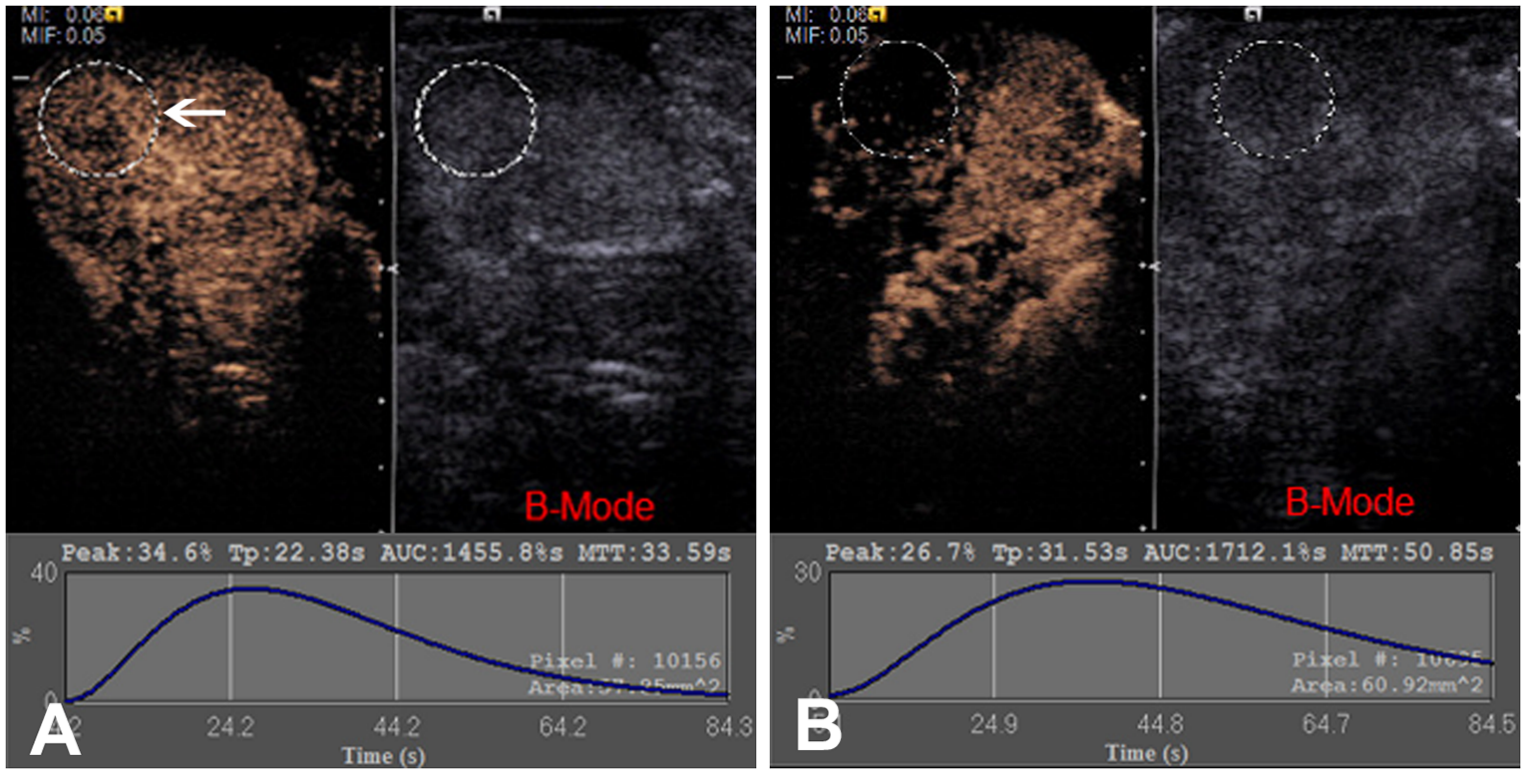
Analysis of the contrast-enhanced ultrasound in microbubble-enhanced ultrasound and prothrombin treated prostates. Interface of the acoustic quantification software showing the contrast-enhanced ultrasound and the 2-D images. (A) A spherical region of interest was set, and a time-intensity curve was analyzed before treatment. (B) In the microbubble-enhanced ultrasound and prothrombin groups, the curves after treatment show that the value of the peak decreased, compared with before treatment.
